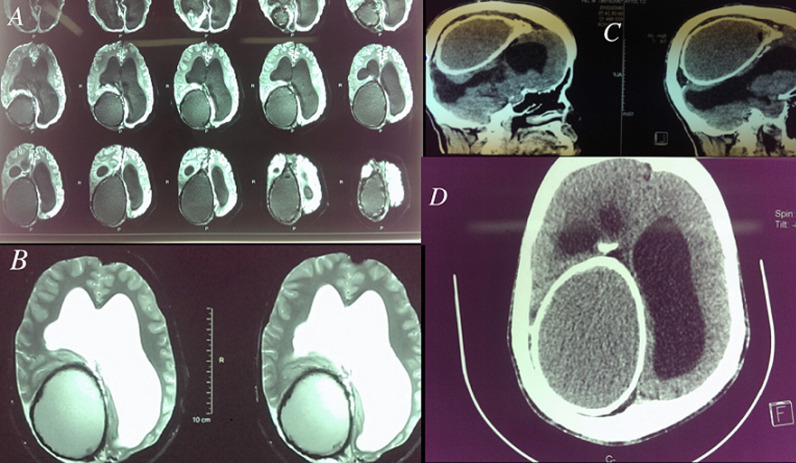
A) FLAIR- weighted cerebral MRI on axial sequences: a sequellar ventriculomegaly without signs of transependymal resorption, in addition to an intracerebral, right occipital, oval formation with a complete calcification of the wall; B) T2- weighted cerebral MRI on axial sequences: the oval formation seems to be extra axial with a fluid density, but different from the CSF signal; C) cerebral CT scan on parenchyma window and sagittal sequences: despite the large volume of the oval formation, there is no perilesional edema, in favour of the age of evolution; D) cerebral CT scan on parenchyma window and axial sequences: a bone bulge adjacent to the calcified cyst; the proximal tip of the catheter of VPS Shunt is seen near the cyst
